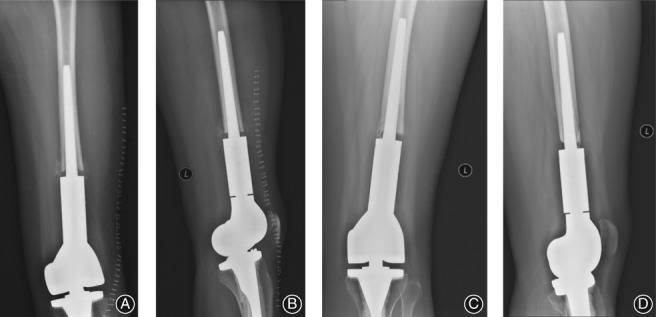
Biologic fixation of a distal femoral prosthesis in a 24‐year‐old woman following resection of an osteosarcoma. Frontal (A) and lateral (B) radiographs showing an adequate contact between the prosthetic stem and the medial side of the cortical bone in the medullary cavity, with a visible 1–2 mm space between the prosthesis stem and the bone. Repeat frontal (C) and lateral (D) radiographs obtained 54 months post‐implantation, showing good contact between the prosthesis and the medullary cavity and satisfactory fixation, with the space between the bone and the prosthesis being maintained, with formation of a bony bridge.
